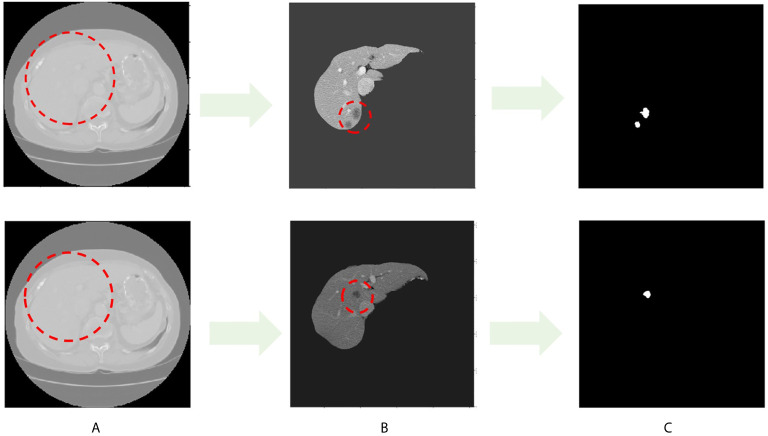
CT image sample with liver and liver tumors. (A) Images in LITS dataset. (B) Liver image. (C) Tumor label.
